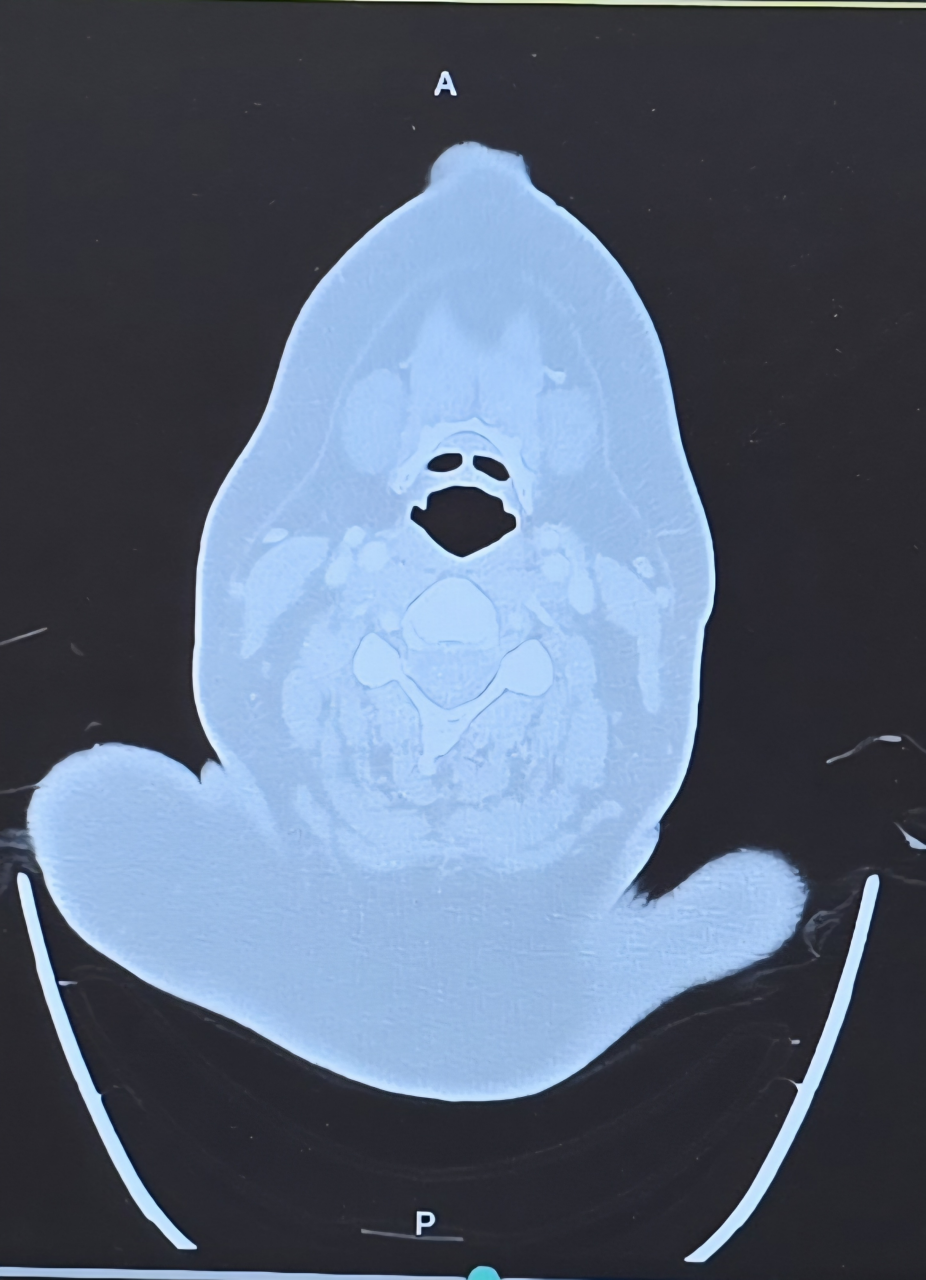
Axial CT image of the neck at the level of the larynx demonstrating normal airway patency and surrounding soft tissue structures, with no evidence of mass lesion, airway narrowing, or structural abnormality to explain chronic cough. CT: computed tomography
